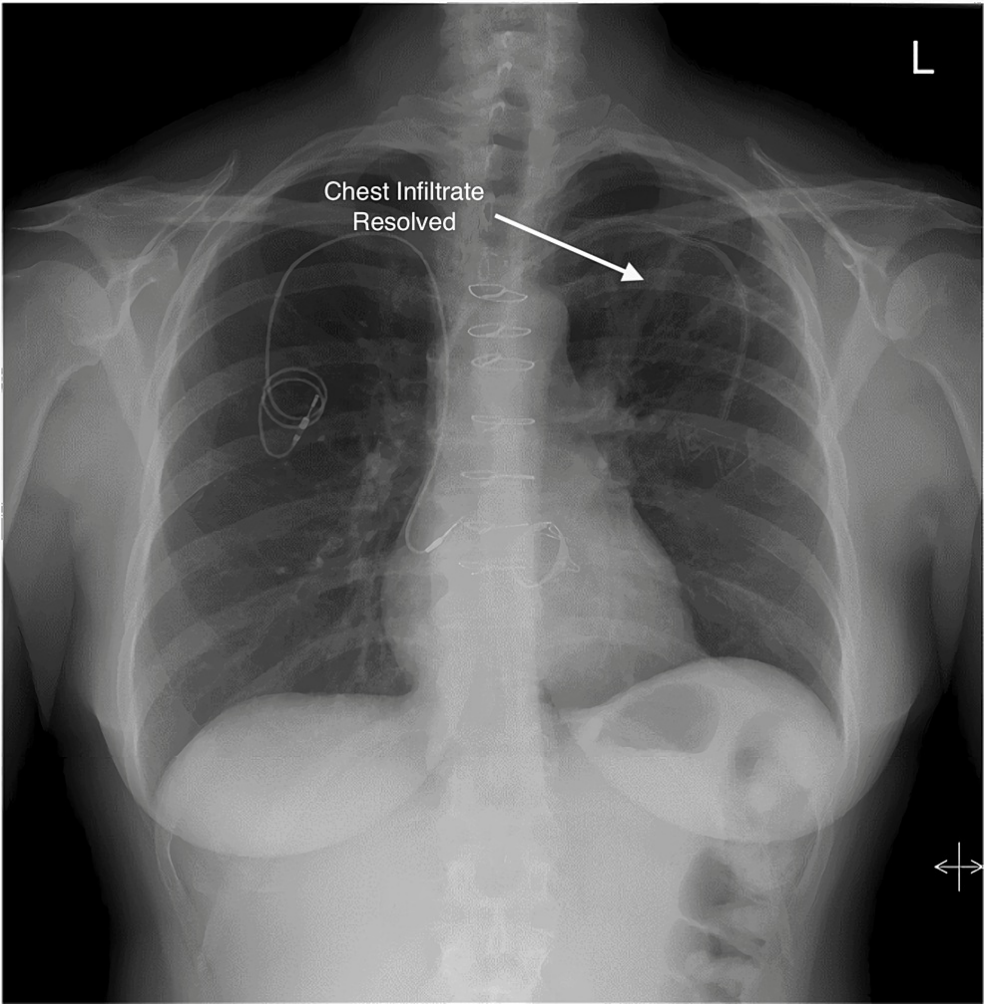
Chest X-ray demonstrating resolution of the left upper lobe pulmonary infiltrate.
